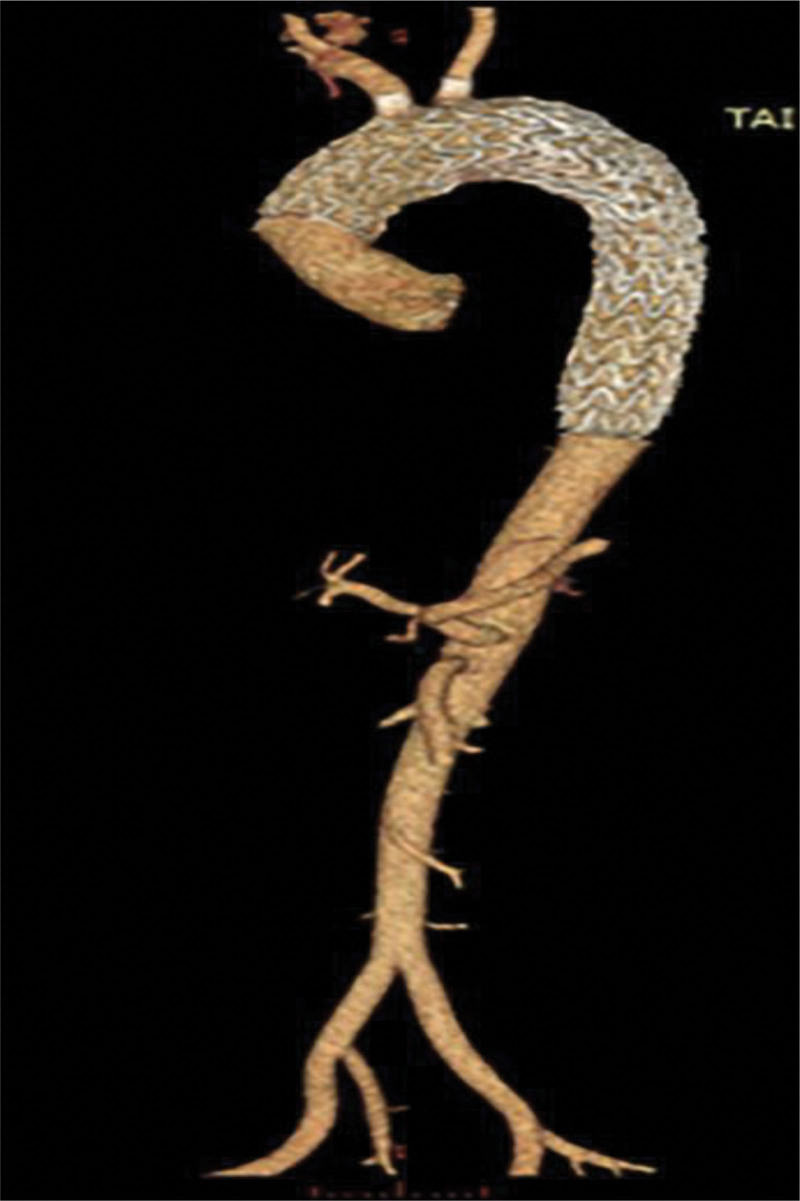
The 3-yr follow-up CTA. CTA shows that there was no perivalvular leakage in the aortic root or pseudoaneurysm in the anastomosis of the ascending aorta. CTA = computed tomography angiography.
